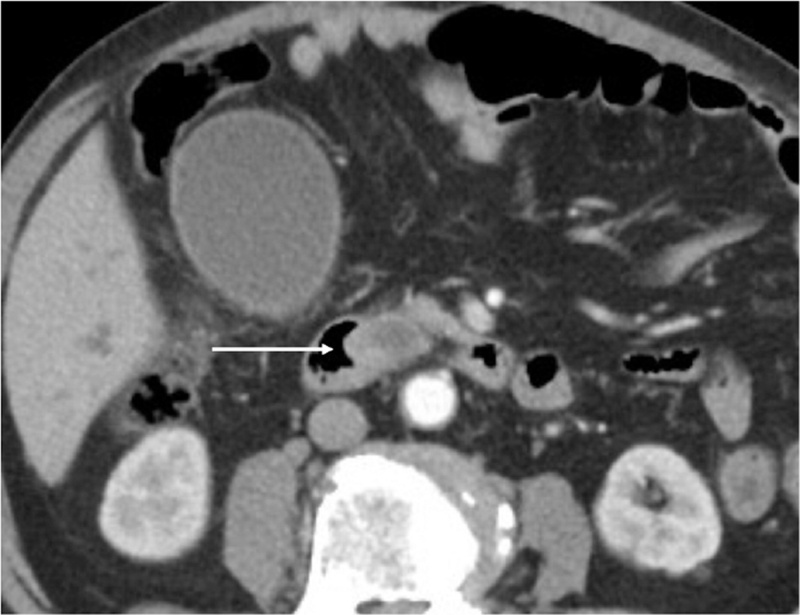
Arrow: CT revealed a 2.16 × 1.92 cm enhancing nodule over ampulla vater region. CT = computed tomography.
